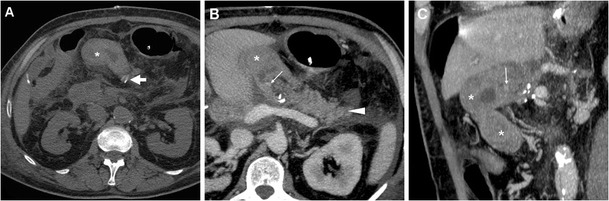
Extraluminal haemorrhage in a 70-year-old man after PD for pT3N1 carcinoma of the Vaterian ampulla, who experienced acute abdominal pain on postoperative day 3: precontrast (a) showed hyperattenuating blood (*) extending ventrally from the surgical bed. Contrast-enhanced CT with thin-slab MIP reconstructions (b, c) showed a tiny focus of CM extravasation (*thin arrows*) consistent with active bleeding. Note normal-appearing PR, drainage tube (*thick arrows*). Relaparotomy was required to stop bleeding from a small artery at the site of pancreatic resection
